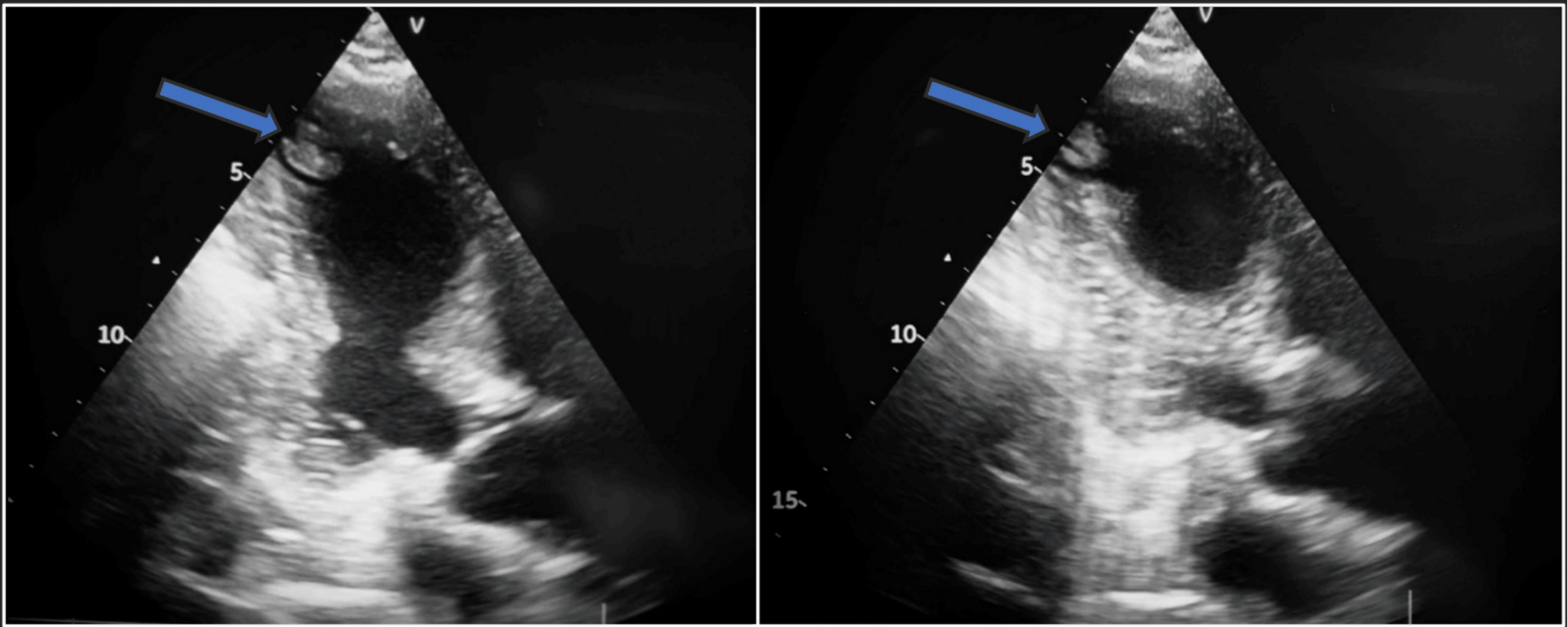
Bedside echocardiogram. Apical three-chamber view showing a thrombus on the apex of the left ventricle.
